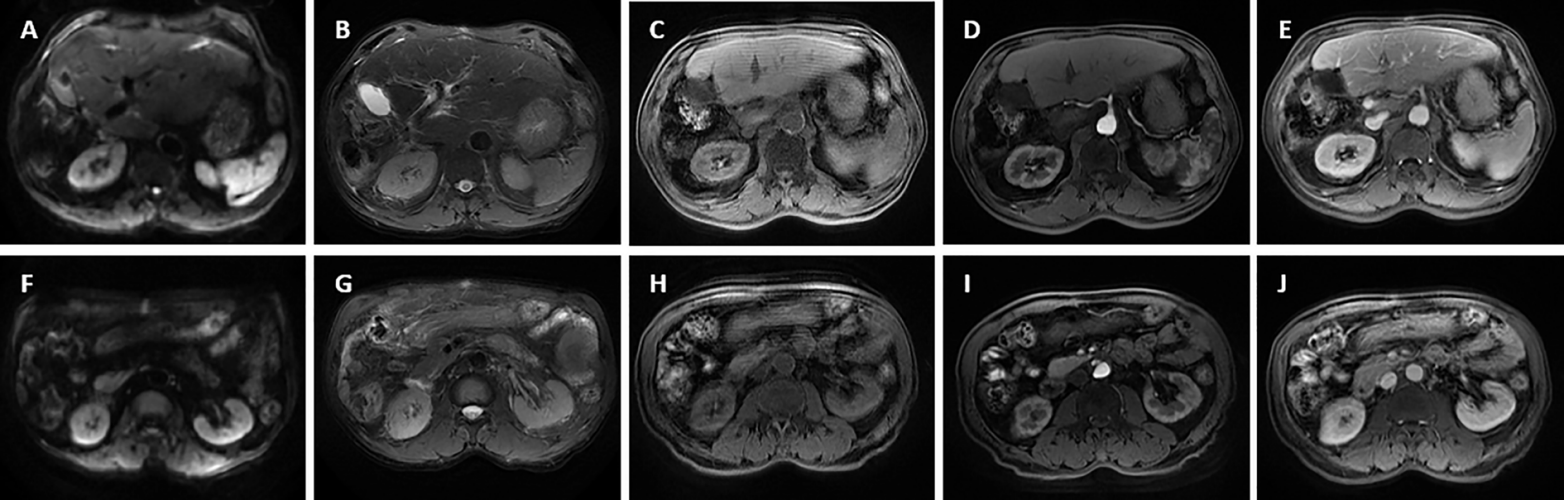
Magnetic resonance imaging was performed for the patient after four cycles of postoperative adjuvant chemotherapy. The tumor has disappeared without recurrence. (A, F) Diffusion-weighted imaging (DWI); (B, G) T2-weighted imaging; (C, H) T1-weighted imaging; (D, I) The arterial phase; (E, J) The delayed phase.
